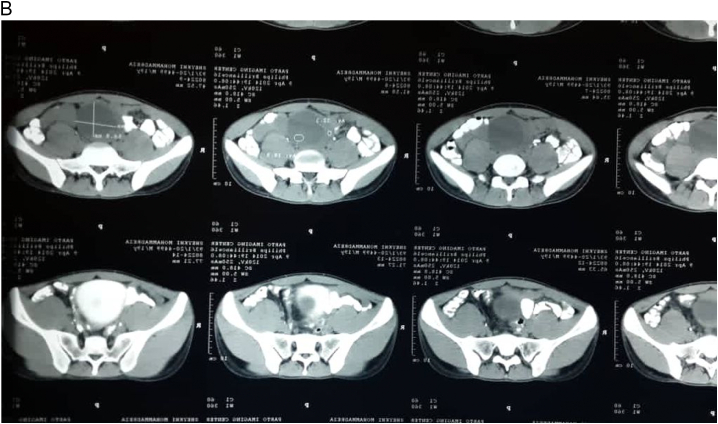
Computed tomography (CT) scan of the abdomen, Iv contrast was administered orally and intravenously. A) CT scan demonstrating a round lesion with a defined margin. B) The lesion puts pressure on the bladder's posterior left side.
